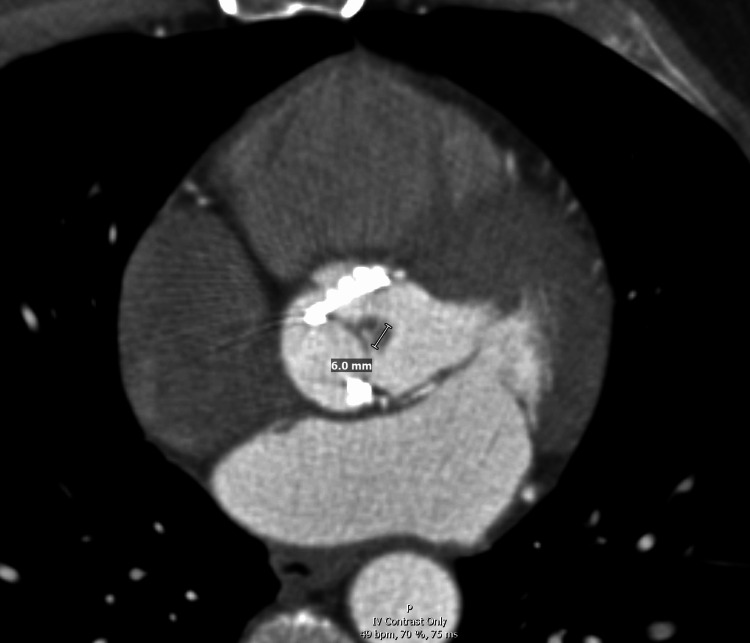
Coronary computed tomography angiography (CTA) showing a 6 mm vegetation on the prosthetic valve.
